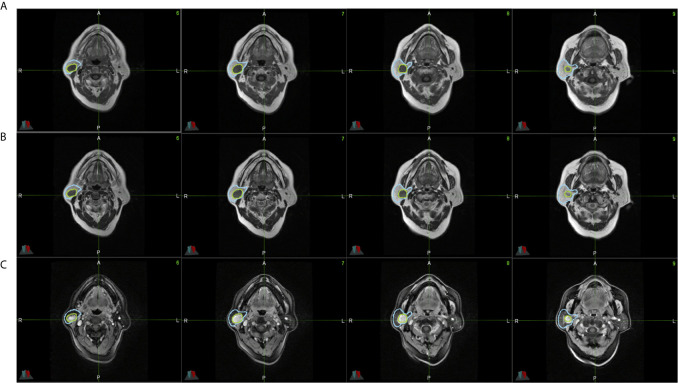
All parotid gland and tumor ROIs from a single patient’s lesion on MR images. (A) shows T1-weighted MR images. (B) shows CE-T1-weighted MR images. (C) shows T2-weighted MR images. The blue region is the parotid gland, and the green region is the tumor.
